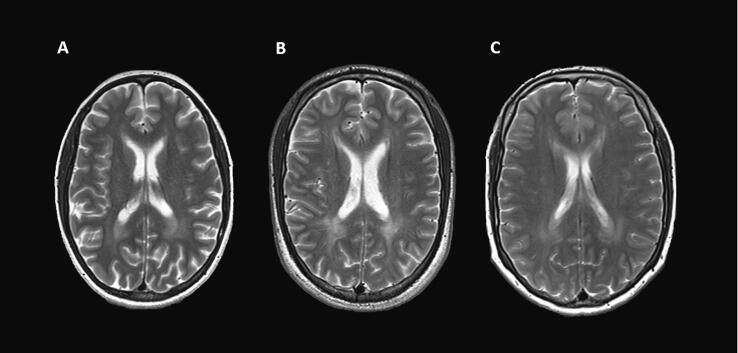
Typical periventricular white matter lesions in adults with PKU. *Note.* Axial slices of T2-weighted images showing typical white matter hyperintensities in three adult patients with PKU (A-C). These abnormalities are most frequently seen in the periventricular white matter of the brain and appear as increased signal (hyperintensities) on T2-weighted images. All patients gave their consent to publish their MR image.
